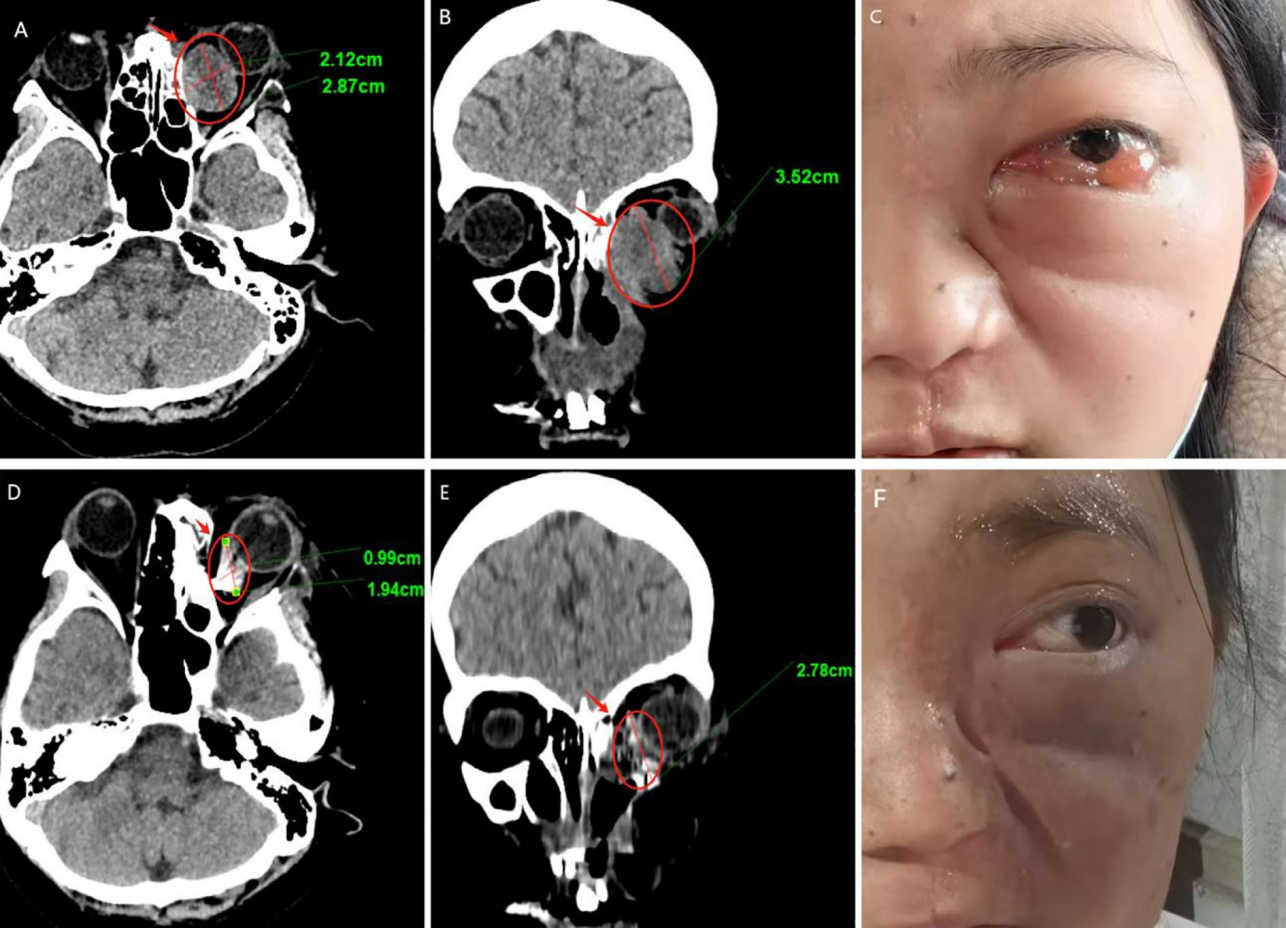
Follow-up results (A–C) Pre-therapeutic imaging series, (D–F) Post-therapeutic imaging series (12-month follow-up) (A, D) CT axial views, (B, E) CT coronal views.
